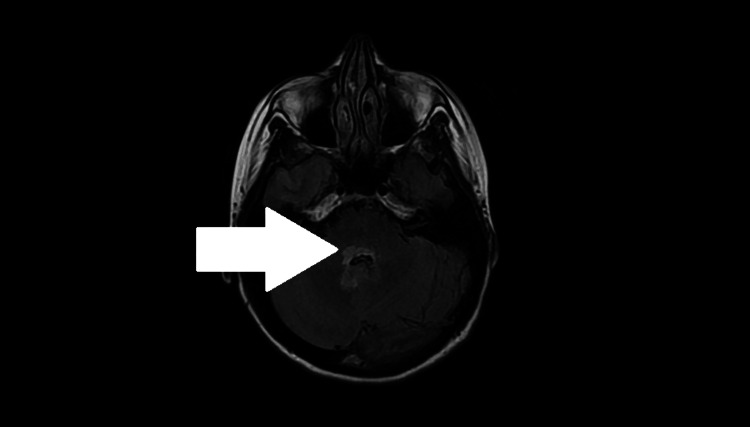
MRI brain FLAIR image showing dorsal pontine lesion with contrast enhancement (White arrow), demonstrating pontine involvement in NMOSD. Axial T2-weighted fluid-attenuated inversion recovery (FLAIR) MRI of the brain reveals a hyperintense lesion within the dorsal pons and cerebellar peduncles, with patchy contrast enhancement (white arrow). These findings correlate with the patient’s cranial nerve deficits, including diplopia and facial weakness, consistent with “eight-and-a-half syndrome.” Brainstem involvement is a recognized feature of NMOSD and helps distinguish it from isolated lupus myelitis.
